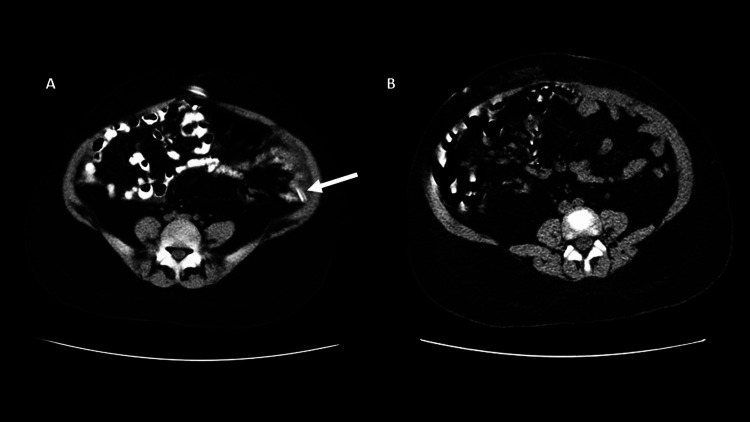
A) Abdominal axial contrast enhanced computed tomography imaging shows the smooth thickened peritoneal covering and numerous peritoneal calcification, bowel dilation with irregular thickening both findings indicating encapsulating sclerosing peritonitis (Tenkhoff catheter is indicated by the arrow). B) Abdominal axial contrast enhanced computed tomography imaging, 6 months later, shows an obvious improvement of the findings as compared to the previous exam, with a great reduction in the number of calcifications and partial recovery of the peritoneum and the bowel.
